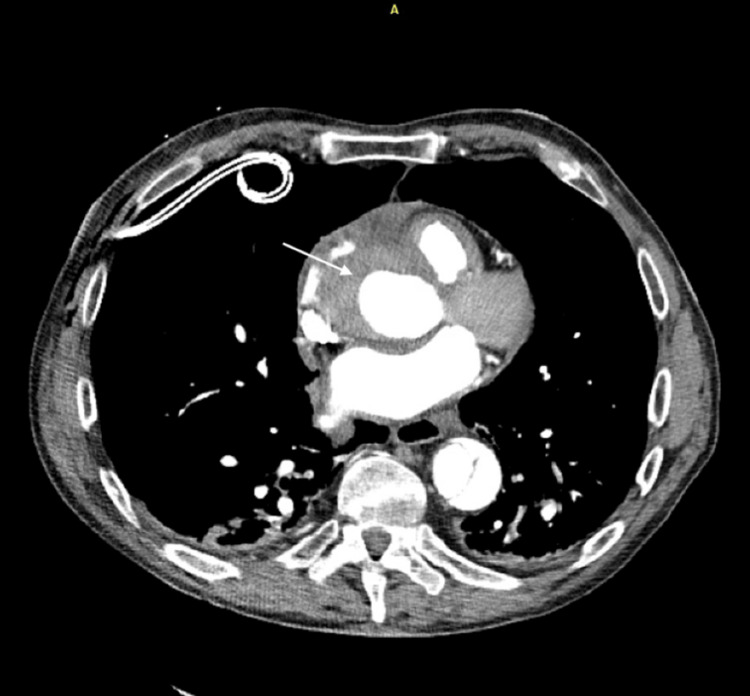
CTA showing type B dissection originating just distal to the left subclavian artery and extending to the infrarenal aorta, a retrograde intramural hematoma involving the ascending aorta and a small pericardial effusion A: anterior
